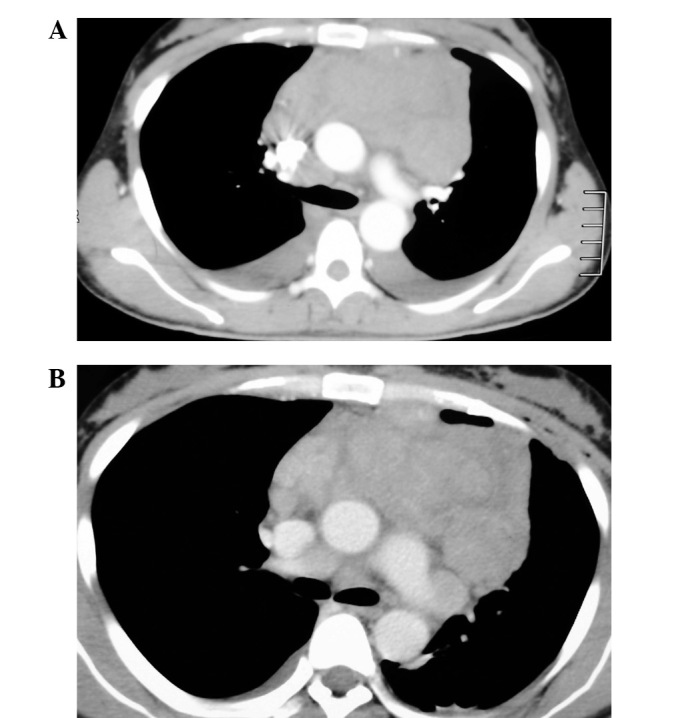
Contrast-enhanced CT scan revealing an upper mediastinal tumor, 9×10 cm in size, adjacent to the arcus aortae, trachea, superior vena cava and left pulmonary artery. The vessels in the mediastinum were compressed and shifted to the lower right, and the trachea became stenotic. There was a small amount of bilateral pleural effusion, but no pericardial effusion was observed. The mass was relatively well encapsulated. Heterogeneous and weak enhancement was observed during the arterial and delayed phases. (A) contract-enhanced image; (B) delayed CT image. CT, computed tomography.
